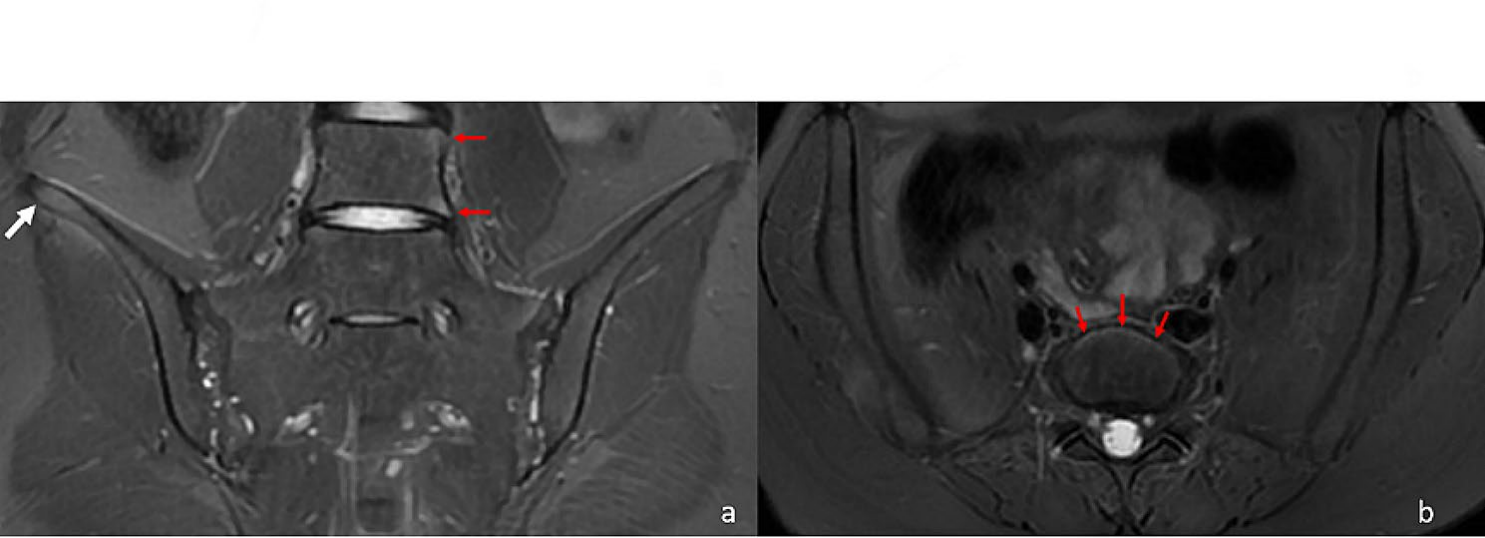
Enthesitis in a 24-year-old female with axial spondyloarthritis. (a) Coronal oblique T2-FS MR image exhibited enthesitis at the right iliac crest and wing, visualized as bone marrow edema and hyperintense signal at the adjacent ligaments (white arrow), as well as anterior longitudinal ligament enthesitis at the L5 vertebra (red arrow). (b) Transverse T2-FS MR image confirmed the presence of anterior longitudinal ligament enthesitis at the L5 vertebra (red arrow)
